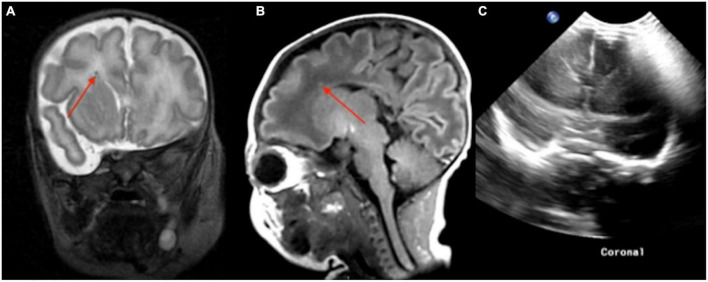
In a routine pre-discharge point-of-care 1-Tesla MRI of a preterm infant, there was a punctate focus of T1 hyperintense (A) and T2 hypointense (B) microbleed in the left anterior periventricular white matter which was not visualized on preceding transcranial ultrasound performed 5 weeks earlier (C).
